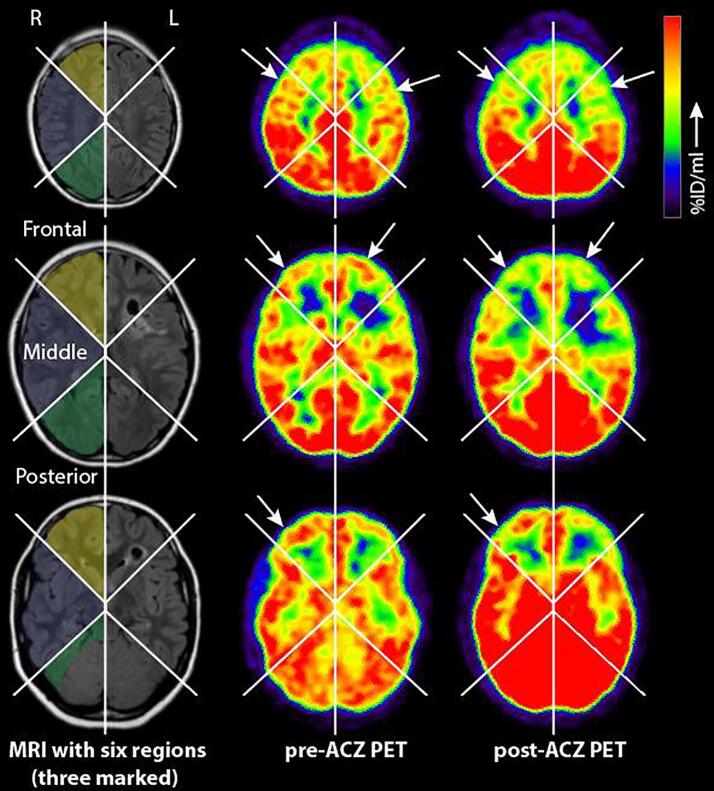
Example of six investigated regions and PET CVR scores. The baseline T2-FLAIR weighted MRI, pre-ACZ and post-ACZ PET images of the same patient shown at three slices (for the PET CVR score all available slices were used). The first column shows the six different regions of interest drawn onto the T2-FLAIR, with the three right regions colored for clarity (yellow: frontal regions, blue: middle regions, red: posterior region. Note: the cerebellum is not part of the posterior region, but is used as a reference). The middle and last columns show the pre- and post-ACZ PET results. The arrows show some examples of (parts of) regions that show vascular steal (negative CVR). The CVR-score of the preoperative scan of this patient is: “steal” in bilateral frontal, and middle territories and “normal reactivity” in bilateral posterior regions. The patient had an infarction left frontally as seen in the T2-flair images. Abbreviations: ACZ: acetazolamide, PET: Positron Emission Tomography, %ID/ml: percentage injected dose per milliliter. (For interpretation of the references to color in this figure legend, the reader is referred to the web version of this article.)
